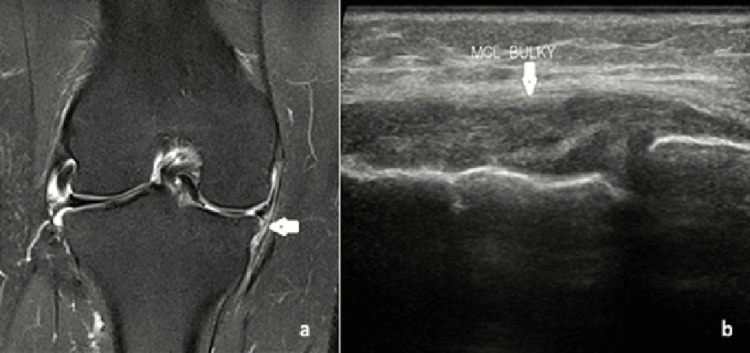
A 30-year-old female patient with a partial tear of the medial collateral ligament (MCL). (a) Coronal PDFS MRI image demonstrating thickening and hyperintensity in the deep fibers of the MCL, suggestive of a partial tear. (b) Corresponding USG image showing a bulky and hypoechoic MCL. PDFS: proton density fat suppression; USG: ultrasonography; MRI: magnetic resonance imaging
